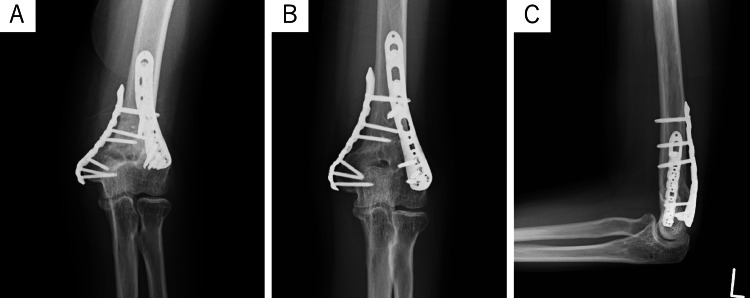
Postoperative radiographs of case one (A) The postoperative radiographs indicated that the left elbow had been corrected with a 10-degree carrying angle (CA). (B, C) The osteotomy site had achieved bone union after six months.
